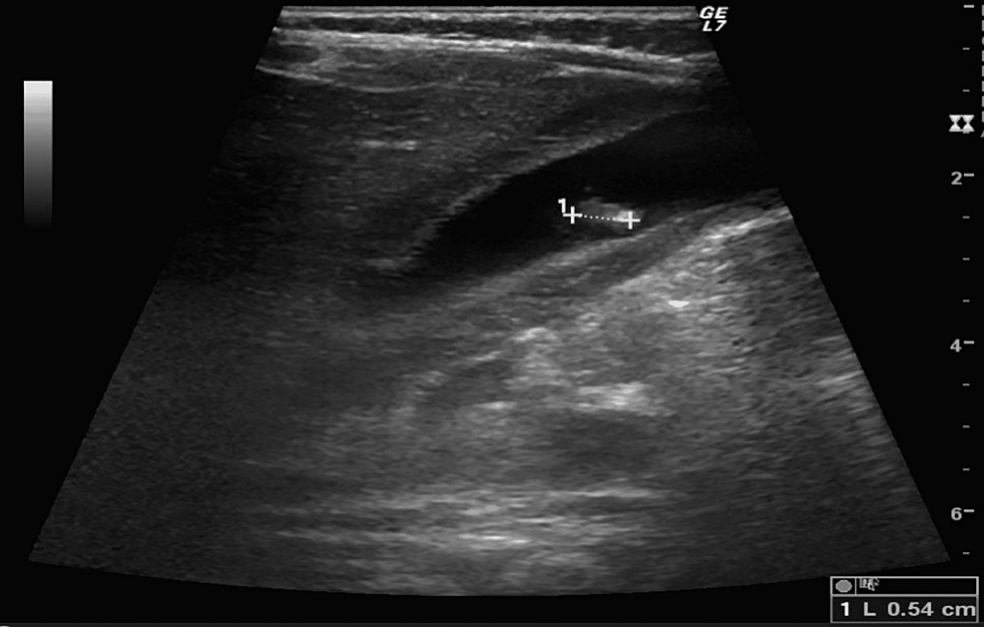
Admission abdominal ultrasound image demonstrating a distended gallbladder with thickened wall and an approximately 6 mm calculus.
